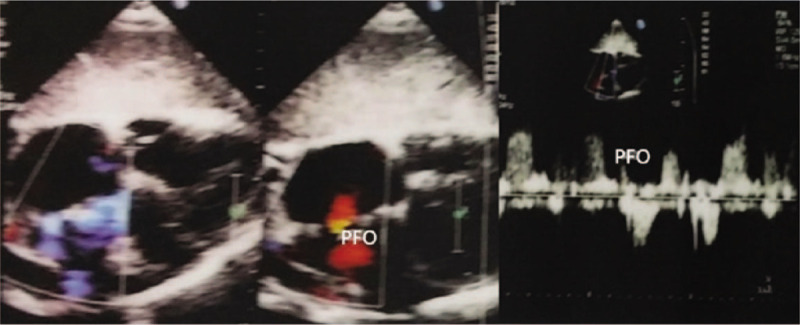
Echocardiography revealed patent foramen ovale, left atrium and right heart dilatated, a large number of tricuspid valve regurgitated.
